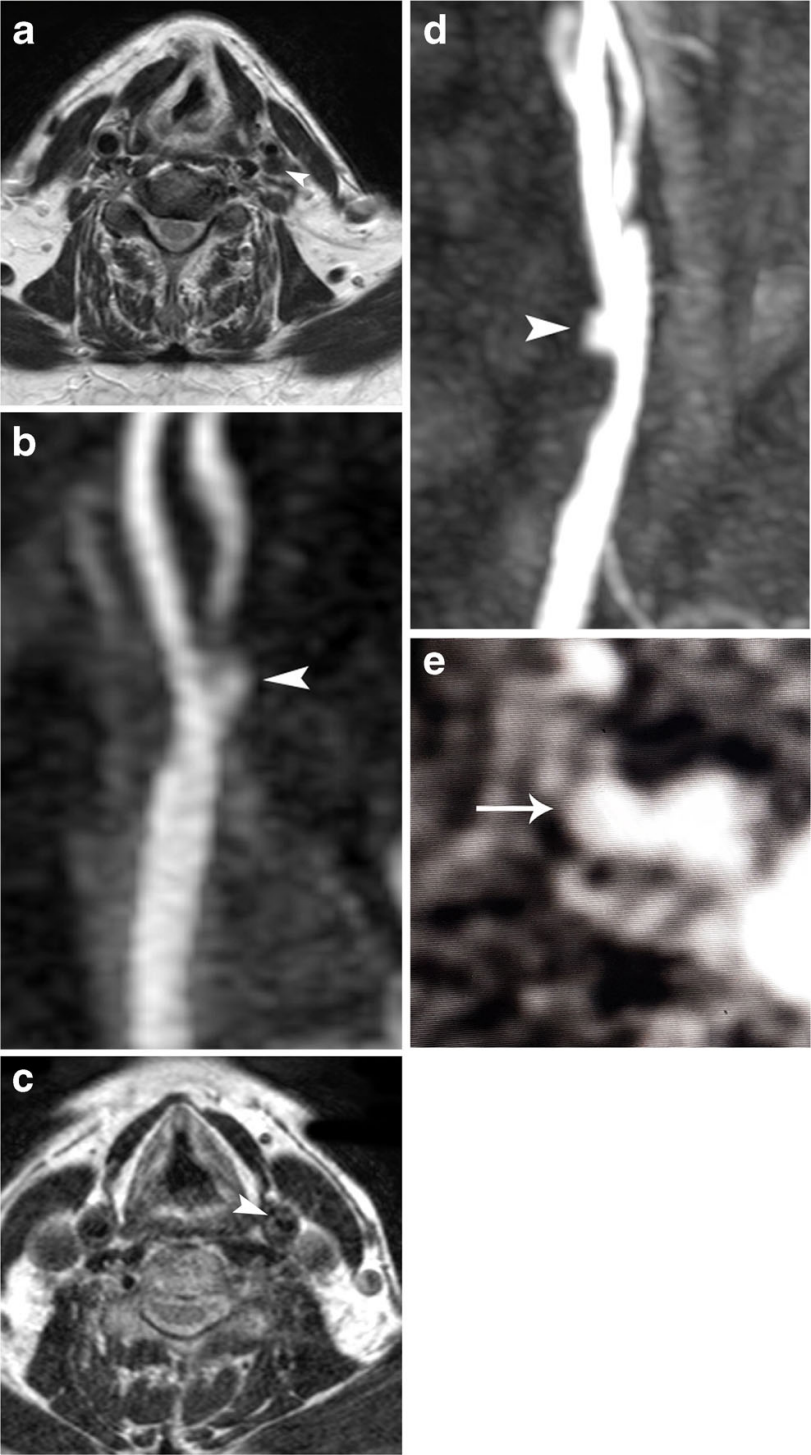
MRA findings in ulcerated carotid plaques. Axial T2-weighted image (a) showing flow void in the vessel lumen and slightly higher signal intensity within the origin of internal carotid artery, possibly representing a stenotic plaque (*arrowhead*). Contrast-enhanced MRA (b) identifying a type 3 ulcerated carotid plaque (arrowhead, same patient with Fig. 4d). Axial T2-weighted image (c) showing a projection of the luminal flow void within a high-signal intensity area, consistent with an ulcerated fatty plaque (*arrowhead*). Contrast-enhanced MRA (d) confirming the presence of a type 1a ulceration (arrowhead, same patient as in Fig. 4a). Axial contrast-enhanced T1-weighted image confirming the presence of contrast material within the ulcer’s cavity (*arrow*) (e)
